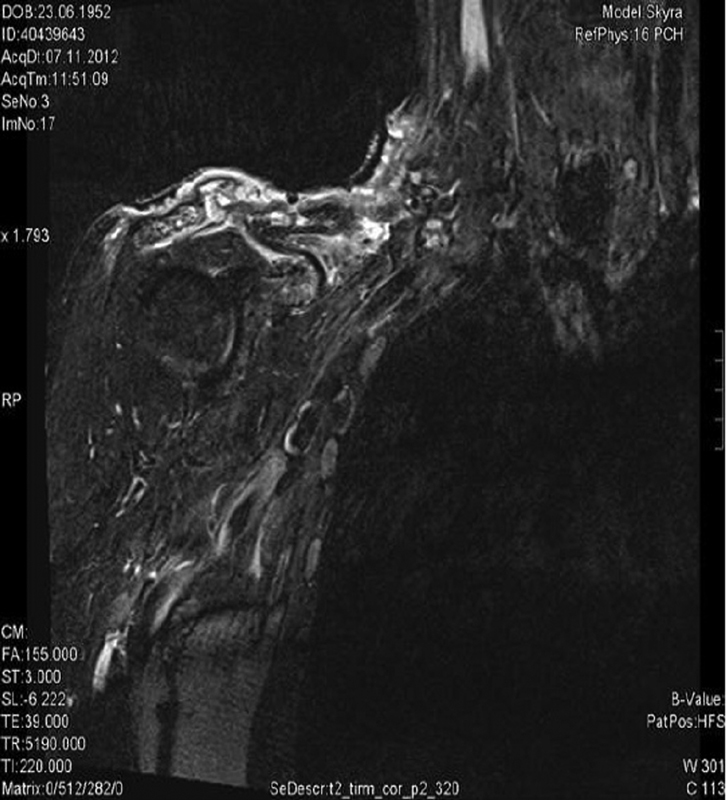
Preoperative magnetic resonance imaging (MRI) scan of the right shoulder for tumor staging. Large destructive basal cell carcinoma in the right shoulder region (TIRM sequence, T2, vertical axis). Complete destruction of the lateral end of the clavicle with surrounding soft tissue and trapezius, subclavian, and supraspinatus muscles.
